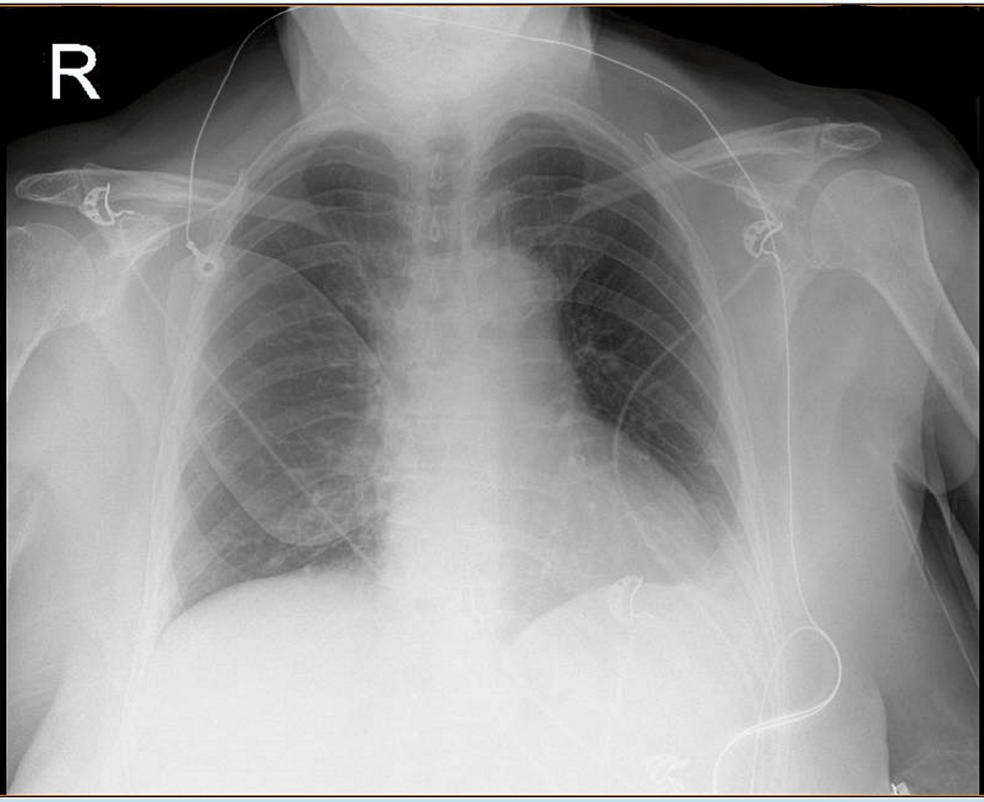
Chest X-ray Chest X-ray with defibrillator pads, no feature of pulmonary oedema, consolidation or pleural effusions.
